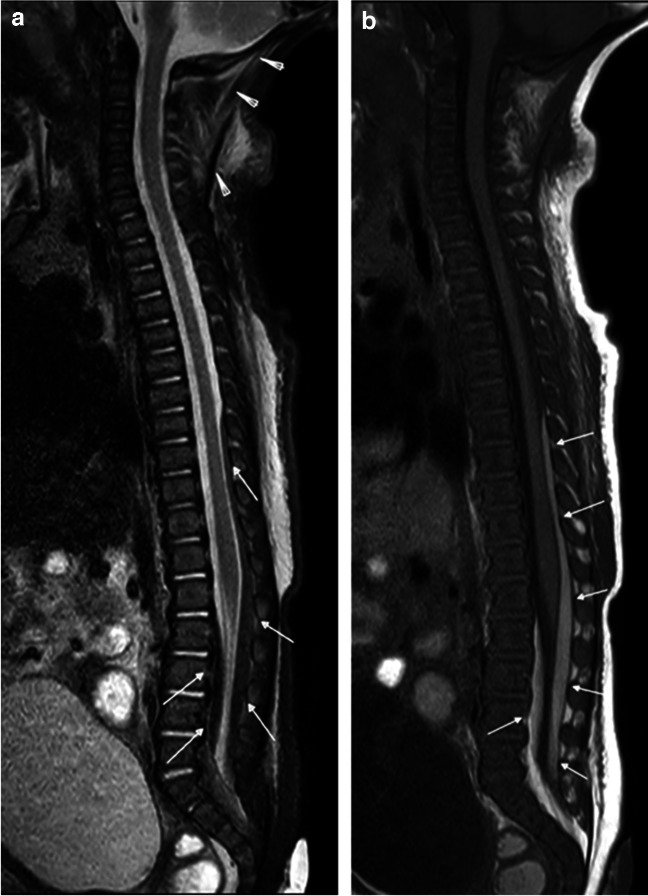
Three-month-old girl presenting with seizure, vomiting, and scalp swelling. Sagittal STIR (a) and T1-weighted (b) MR images of the total spine demonstrate posterior ligamentous complex injury with extensive edema in the nuchal ligament (*arrowheads*), as well the atlanto-occipital, atlanto-axial, and cervical interspinous ligaments. Extensive edema in the subcutaneous soft tissues of the suboccipital region and dorsal soft tissues overlying the thoracic spine. There is also thoracolumbar SDH (*white arrows*) which is T2-hypointense and T1-hyperintense. Additional work-up of this patient (not shown) revealed findings of AHT with mixed attenuation SDH and HII, calvarial fractures, multiple healing rib fractures, and a right femur metaphyseal corner fracture
